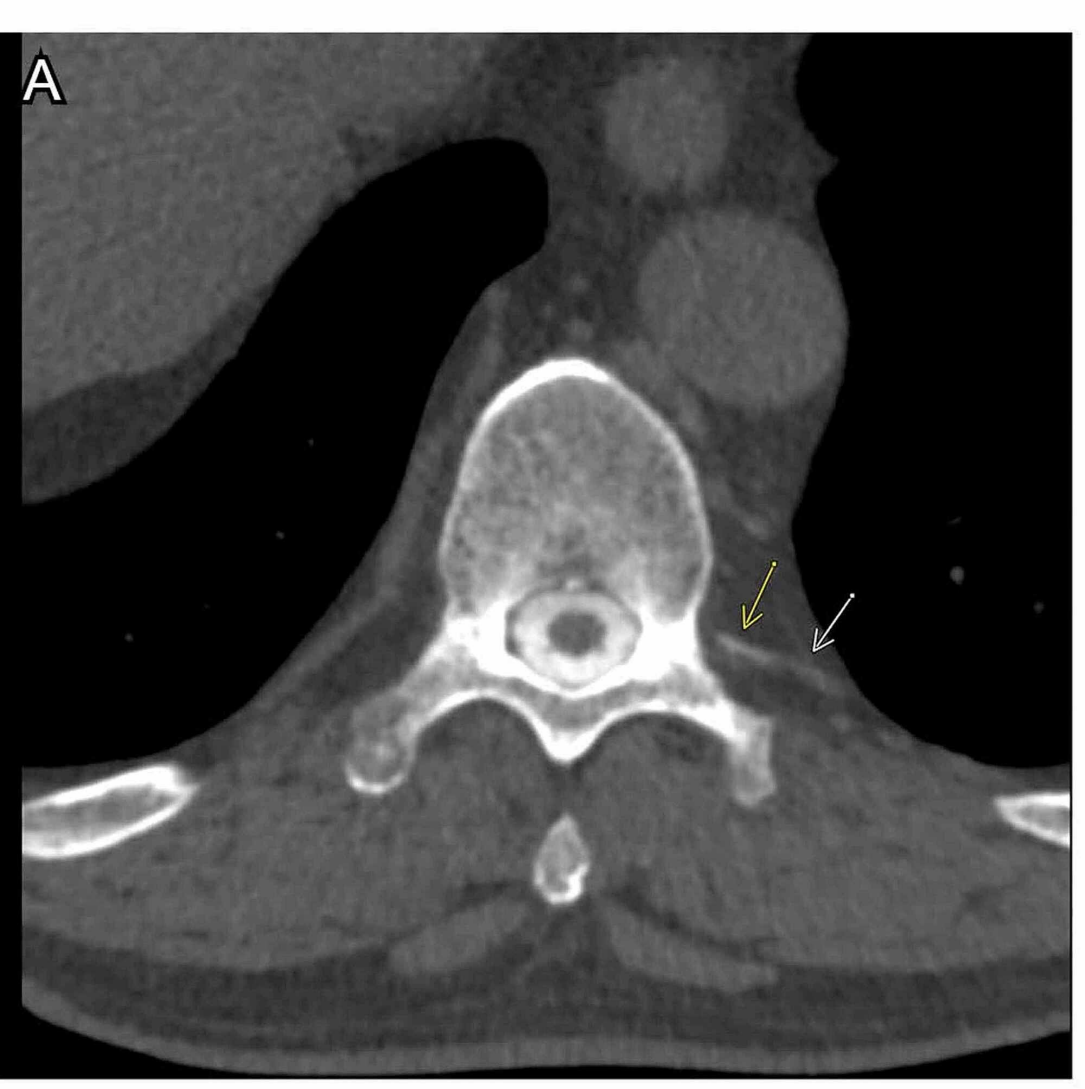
CT myelogram. Axial CT thoracic myelogram showing hyperdense paraspinal vein consistent with CSF-venous fistula arising from left T10-T11 nerve root. CSF: cerebrospinal fluid.
